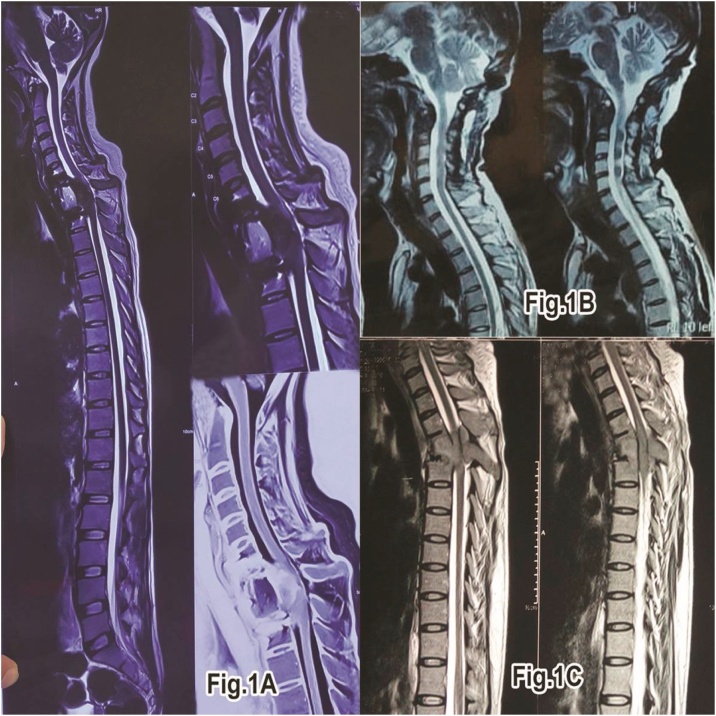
Pre-operative MRI images saggital section showing the ocation of GCT. A:MRI images showing GCT at C7-D1 region. B:MRI images showing GCT at C2 level. C:MRI images showing GCT at D4 level.
